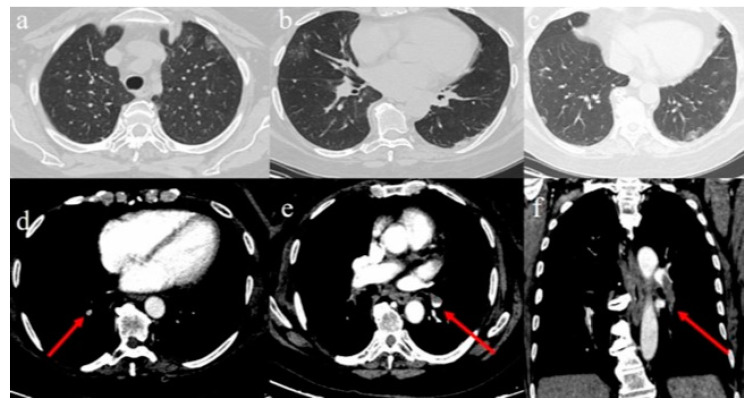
Chest computed tomography (CT) and CT angiography (CTA) of Case 1. Lung window (W: 1600 UH, L: −500 UH) of chest CT scan: findings of COVID-19 pneumonia with peripheral ground-glass opacities in the left superior lobe (a), in the middle lobe, with a posterior peripheral consolidation in the inferior left lobe (b); peripheral ground-glass opacities in the inferior lobes (c). Mediastinal window (W: 251 UH, L: 45 UH) of CTA scan: filling defect in a right subsegmental pulmonary artery branch for the inferior lobe (red arrow in (d)); an extensive filling defect in the lobar and in a segmentary pulmonary arterial branch for the inferior lobe (red arrow in (e) on the axial plane and in (f) on the coronal plane).
